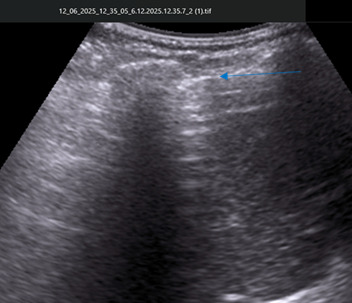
Abdominal point of care ultrasound (POCUS) image, magnified region of interest from Figure 1, same patient and same acoustic window. The blue arrow highlights the enhanced peritoneal stripe and reverberation artifacts along the hepatic margin, consistent with pneumoperitoneum. Supine position; convex transducer 3.6 MHz; longitudinal orientation.
